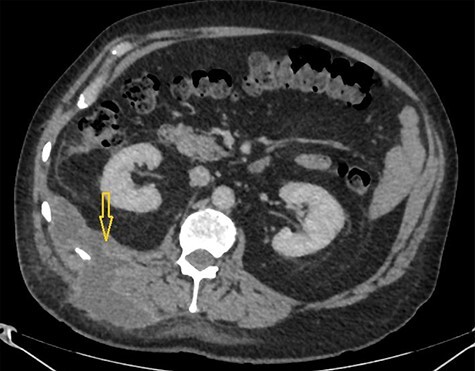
CT scan abdomen demonstrates a retroperitoneal abscess tracking through an intramuscular opening onto skin in the right paraspinal region.
